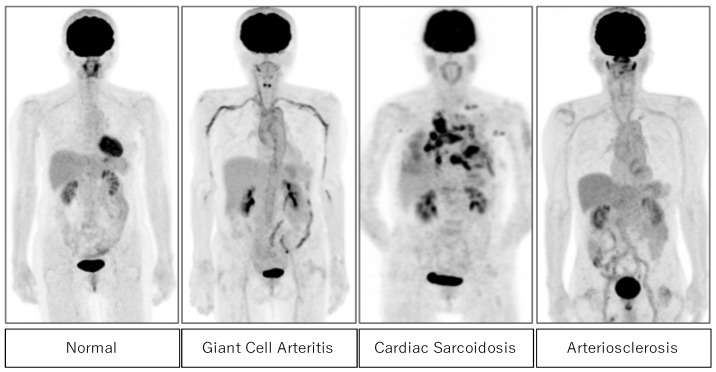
FDG uptake in the normal subject (left) and abnormal FDG uptake in active inflammatory lesions in patients with giant cell arteritis, cardiac sarcoidosis, and arteriosclerosis (right).
